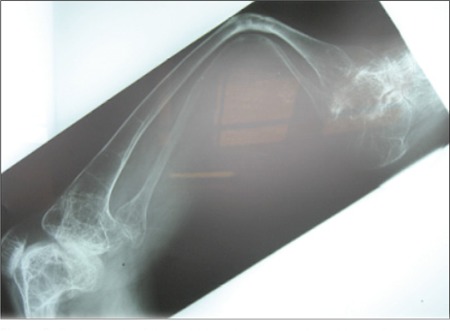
Radiograph of the right leg showing profound loss of bone withsevere bending of the bone shafts and thin cortical layer
